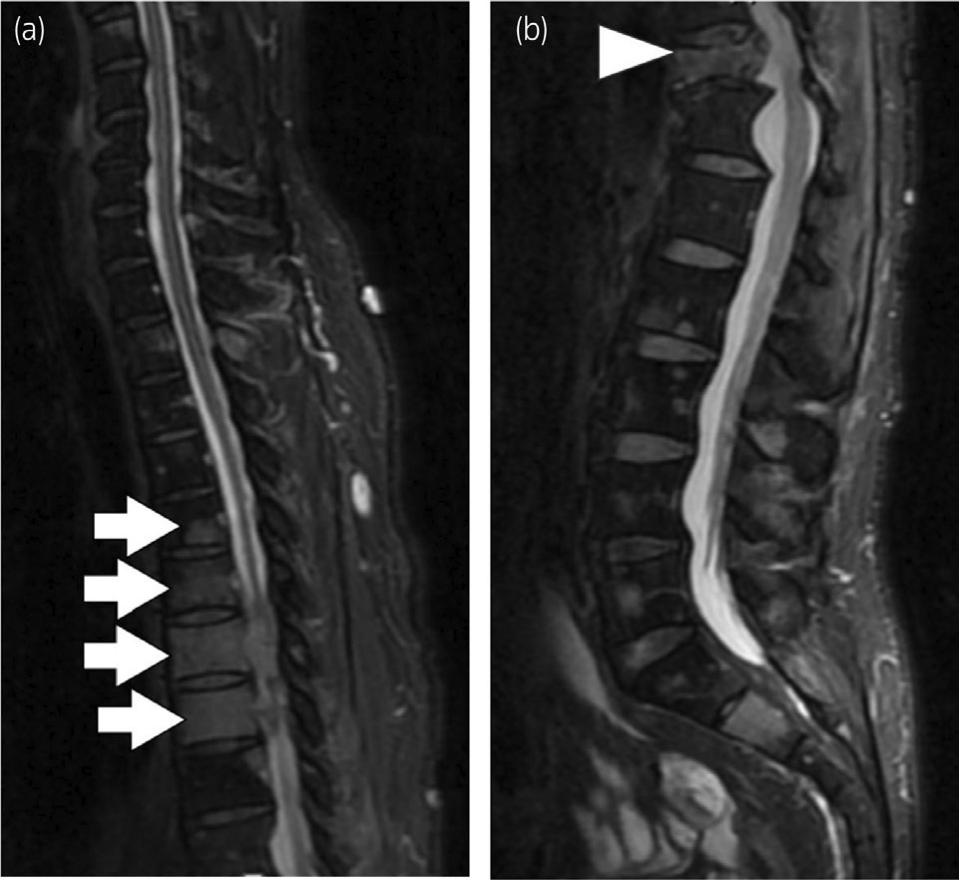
MRI of a patient with onset of lower extremity muscle weakness. Fat‐suppressed T2‐weighted image showing multiple high‐signal areas in the spine. (a) It extends into the spinal canal in the 5th–8th thoracic vertebrae (arrows). (b) A known fracture of the vertebral body in the 11th thoracic vertebra (arrowhead).
